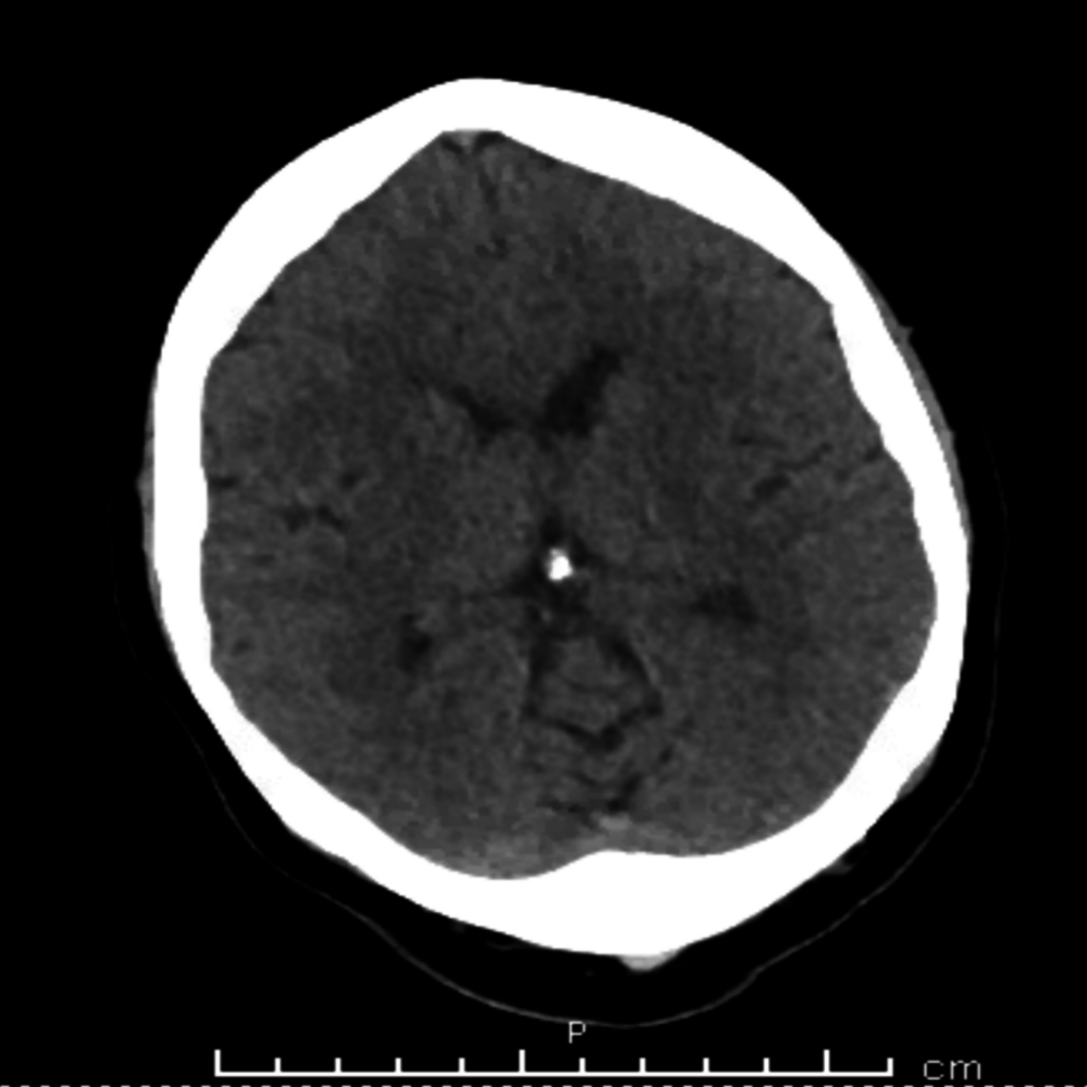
Computed Tomography (CT) Scan of the Head
